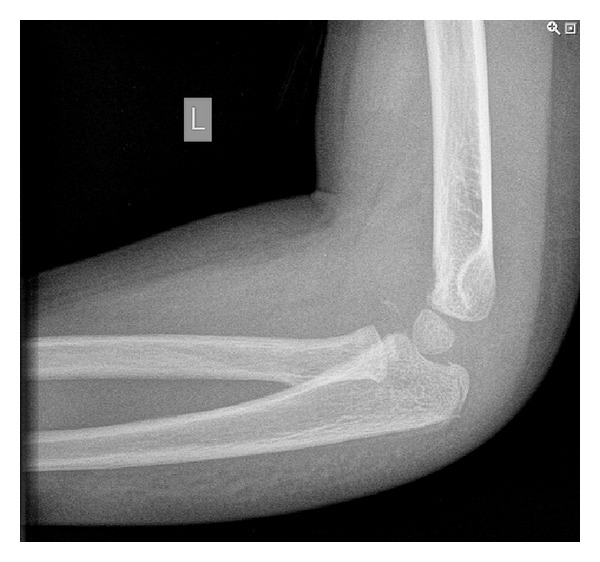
Repeat lateral radiograph of the same elbow twelve days later. The olecranon fracture is more defined.
